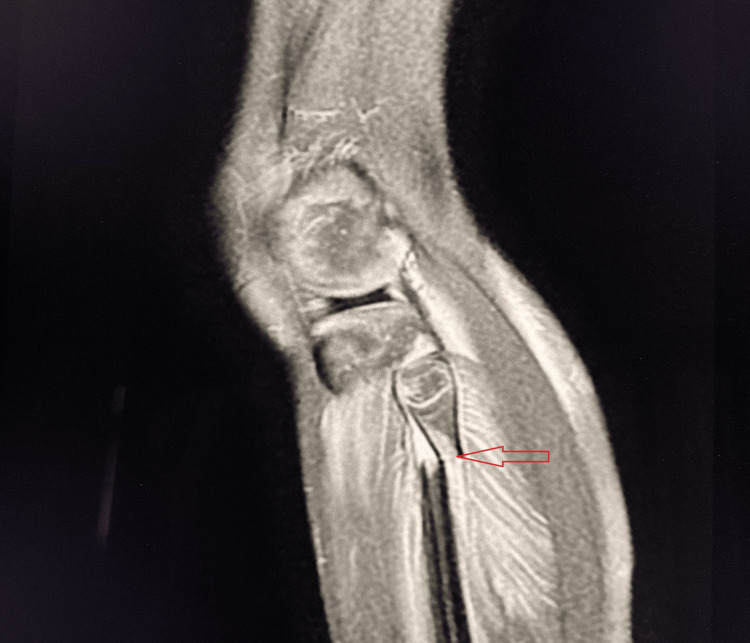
Transverse fracture of fibular neck Sagittal PD-FS MRI demonstrates a transverse fracture of the fibular neck (red arrow) with posterior displacement of the proximal fragment, associated with marrow edema and surrounding muscular edema. PD-FS, proton density fat-suppressed
